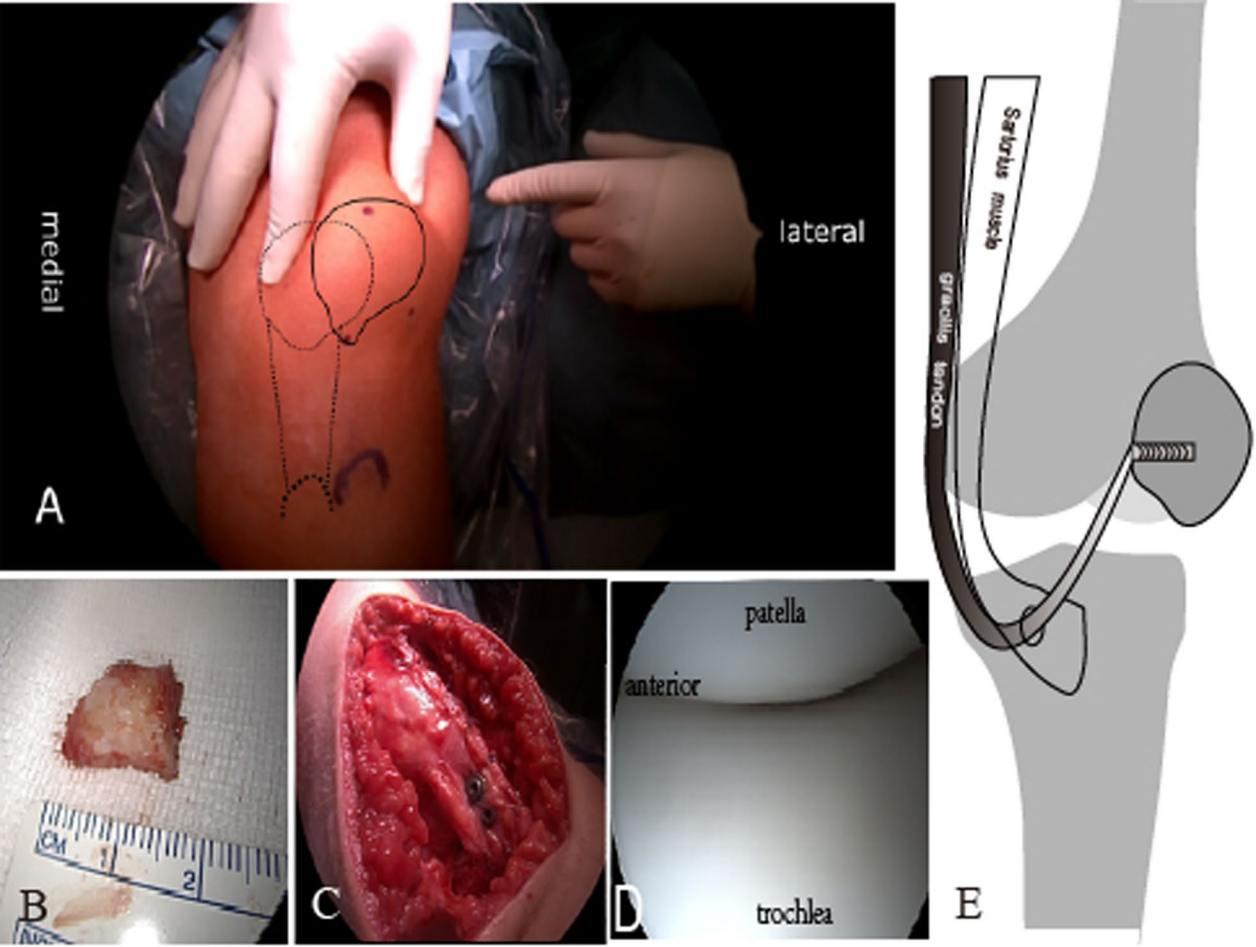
A A postoperative anterior view radiograph taken in the operating room indicated that two screws had cross-fixed the tibial tubercle. B A postoperative lateral radiograph of the left knee, also taken in the operating room, showed a substantial reduction in patellar height; the Caton–Deschamps index had decreased from 1.7 preoperatively to 1 postoperatively. C A lateral radiograph taken at the 2-year follow-up postoperatively still maintained a Caton–Deschamps ratio (a/b) of 1 and an morphology ratio (c/a) of 1.4. D The 2-year postoperative follow-up revealed that the flexion angle was 120° and the extension angle was 0° E
